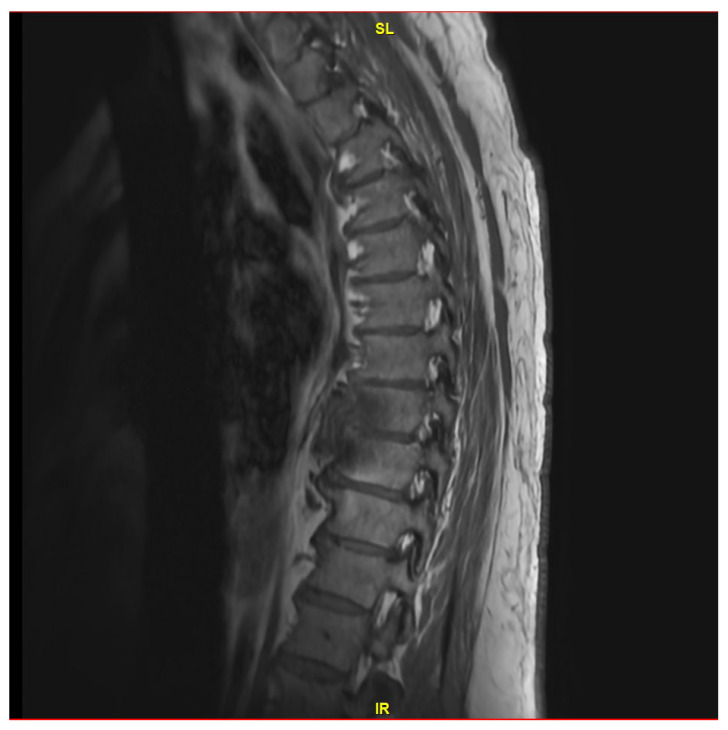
MRI thoracic spine with and without IV contrast revealing small T8 fluid collection and possible diskitis T8-T9.
